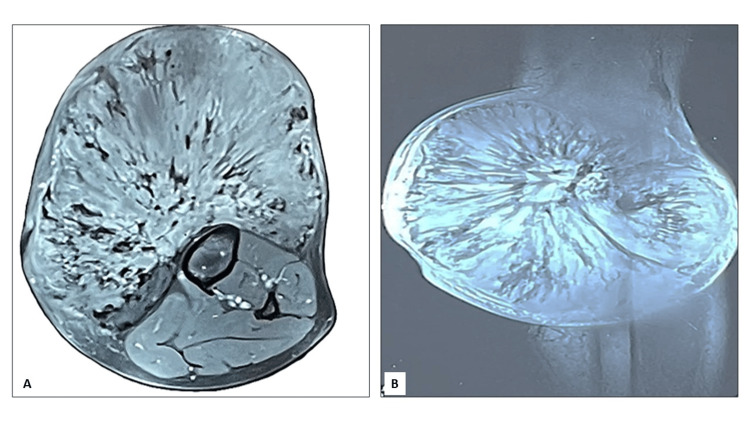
(A) Axial (T1) and (B) coronal (T2) MRI images showing soft tissue mass with bone involving the upper part of the tibia
